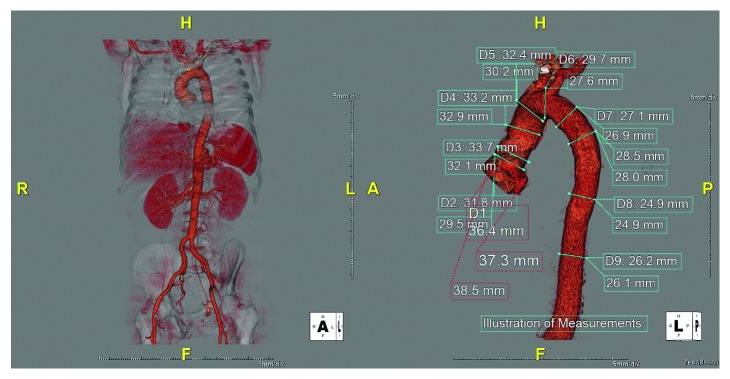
CT angiogram with 3D reconstruction demonstrating aortitis without involvement of adjacent branches.
